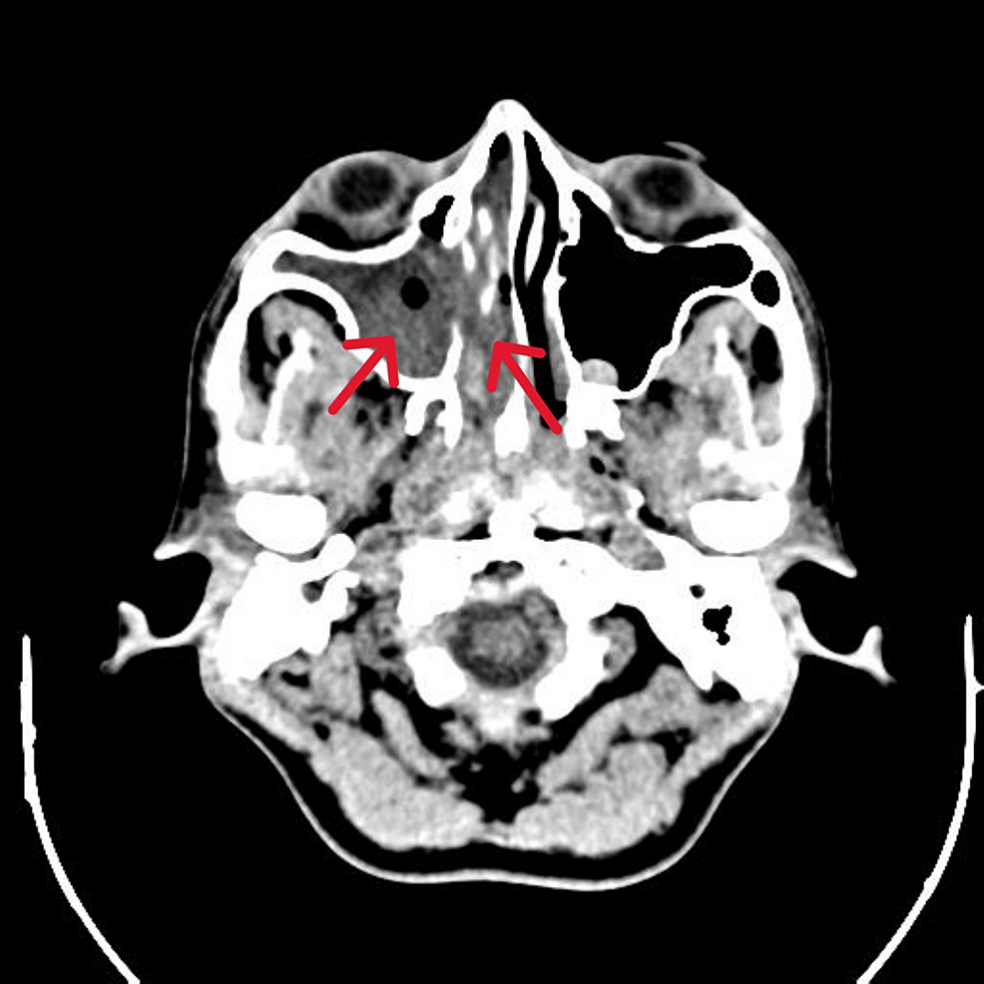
CT paranasal sinus (PNS) axial view shows evidence of paranasal sinus enlargement with remodelling and destruction of sinus walls and nasal turbinates, suggestive of nasopharyngeal angiofibroma (grade 2 A) (red arrow)
